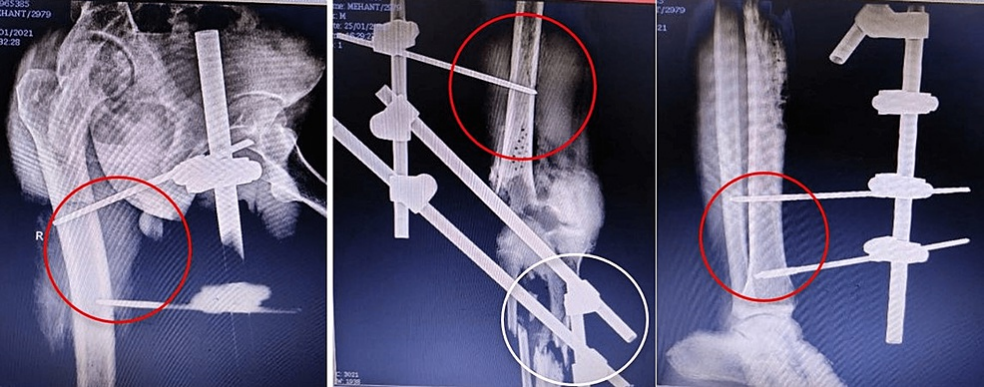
Postoperative X-ray where an external fixator was applied
